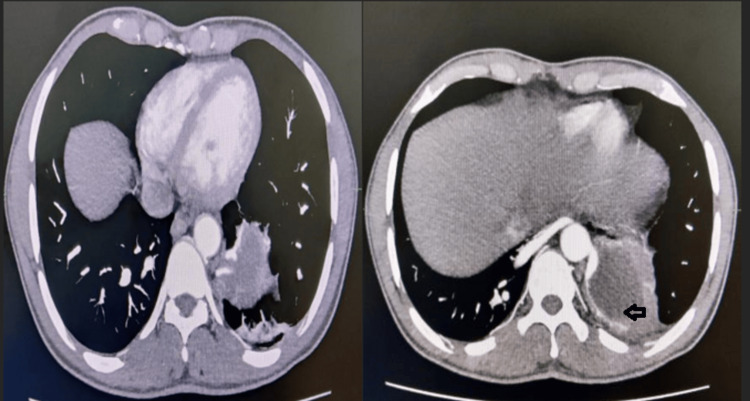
The axial section of the CT angiogram shows the sequestration tissue (arrow).
